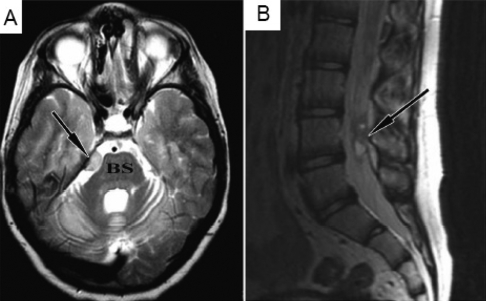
Pre-Operative MRI (A) Brain MRI (T2) demonstrating a lesion (arrow) based on the tentorium, next to the brain stem (BS). (B) Spinal-lumbar MRI (T2) showing intradural lesion (arrow), at the level of the L4 vertebra.
